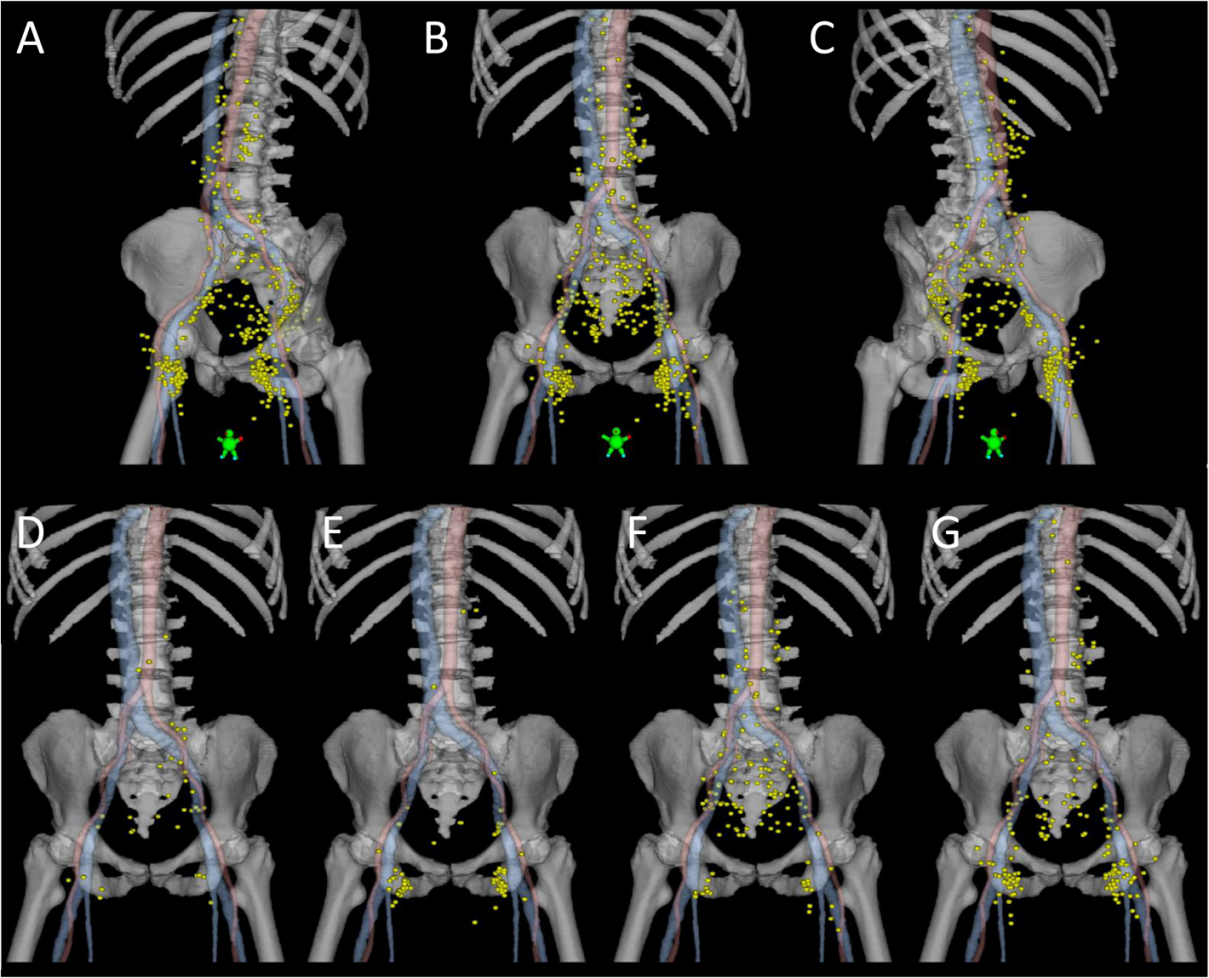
PET-positive lymph nodes (*yellow*) in all patients (a-c), in patients with anal canal tumors (d), anal canal tumors with perianal extension (e), anal canal tumors with rectal extension (f), and anal canal tumors with both perianal and rectal extension (g). Center of lymph node mapped with a 6 mm sphere in a standard anatomy reference CT. *Red*, arteries. *Blue*, veins, including the saphenous vein
